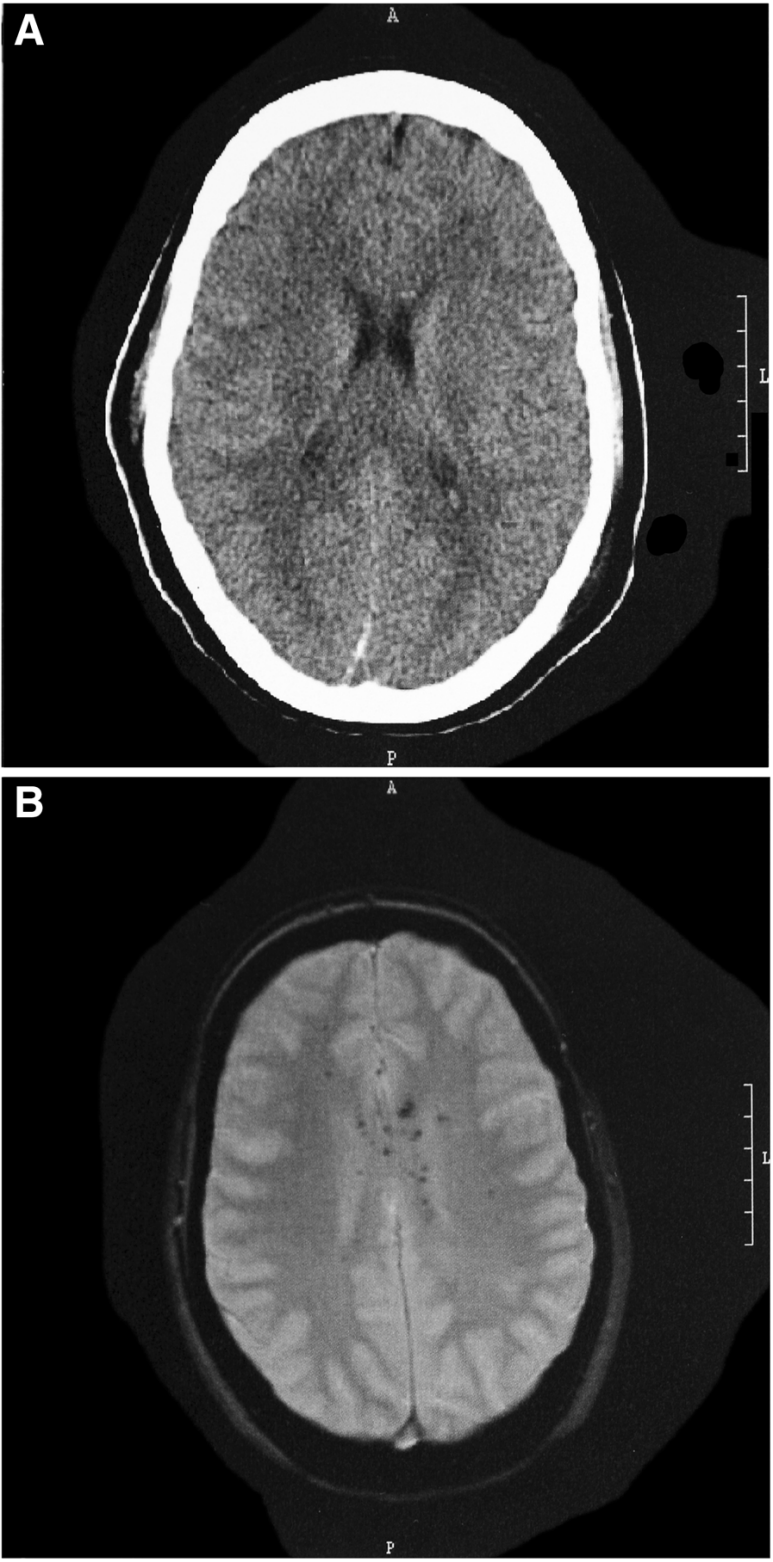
16 y/o patient with traumatic brain injury. A. CT shows no lesion. B. MRI shows multiple disseminated microbleeds in the T2* sequences.
